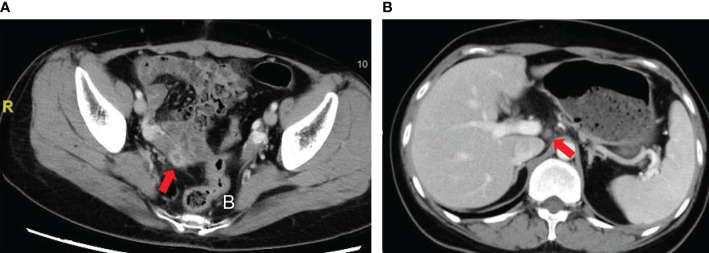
Computed tomography (CT) scan of patient with YST originating from the broad ligament. (A) A 2-cm mass at the right pelvis; (B) Slightly enlarged lymph nodes.
